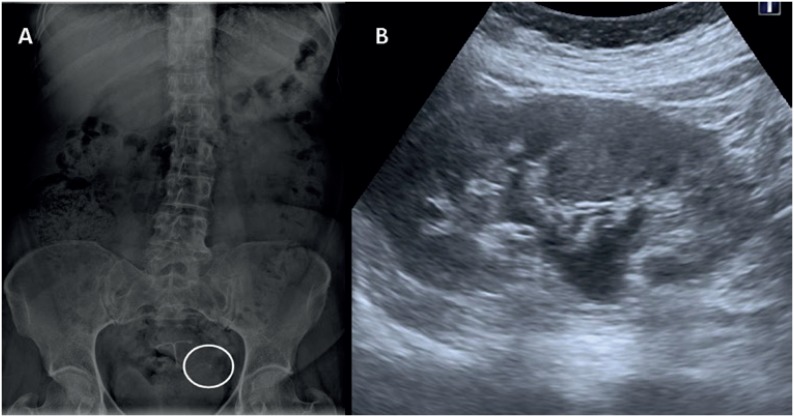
A) Abdominal X-ray with left pelvic calcification (white circle); B) Ultrasound image showing grade II left ureterohydronephrosis.
